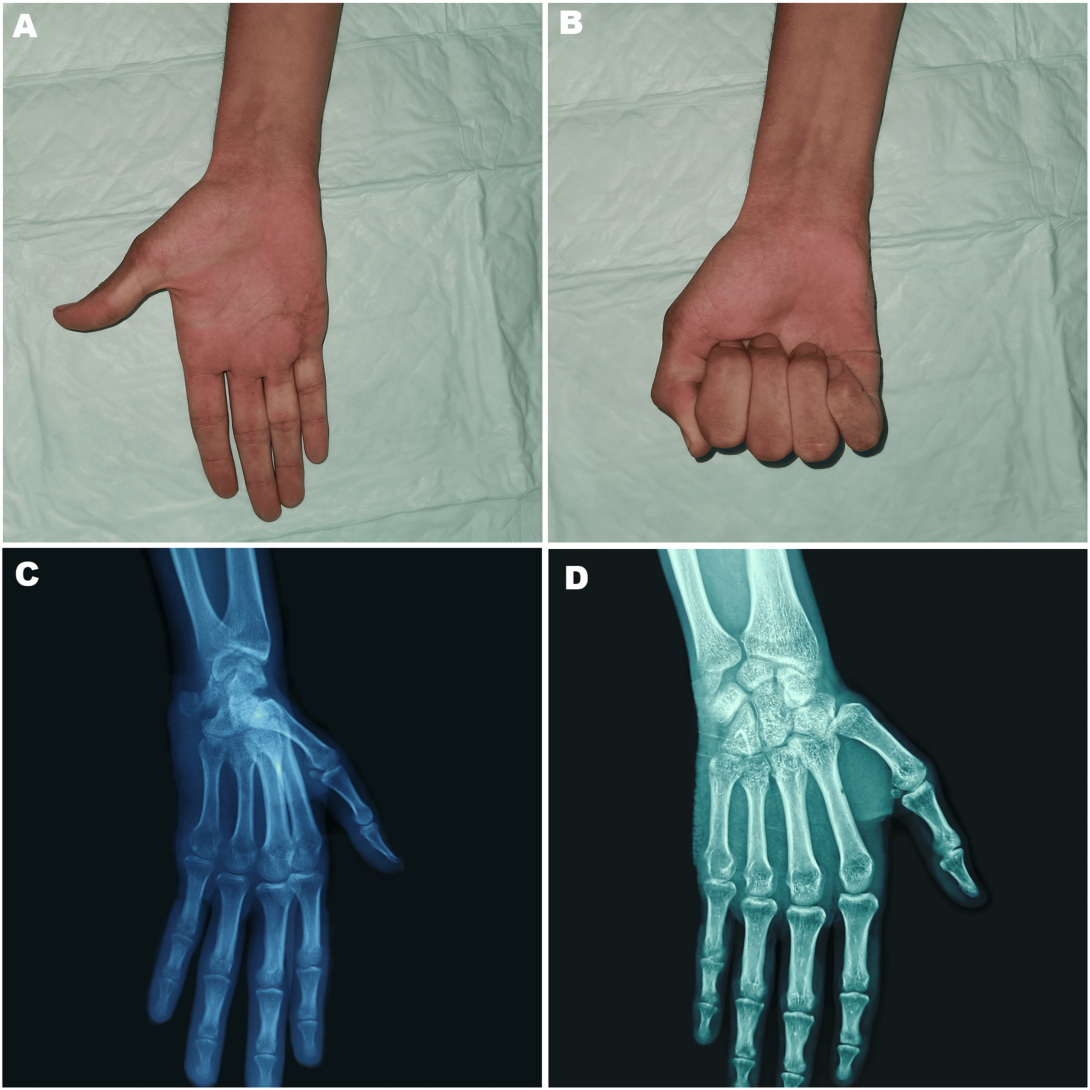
Clinical photographs and X-ray images at the one-year follow-up (A-B) Range of motion was assessed showing (A) extension, (B) flexion; (C-D) No redislocation and no evidence of arthritis
